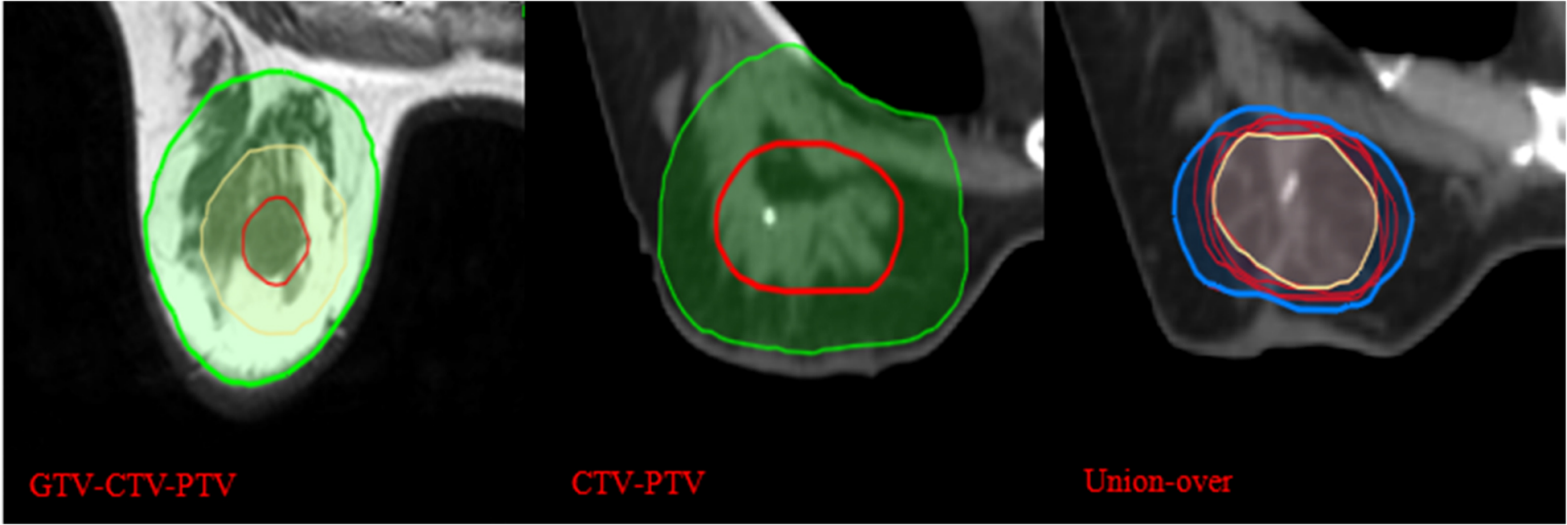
The delineation of target volume based on pre-MRI and post-CT drawn by one radiation oncologist, and the common and encompassing volumes of CTV drawn by 5 observers
